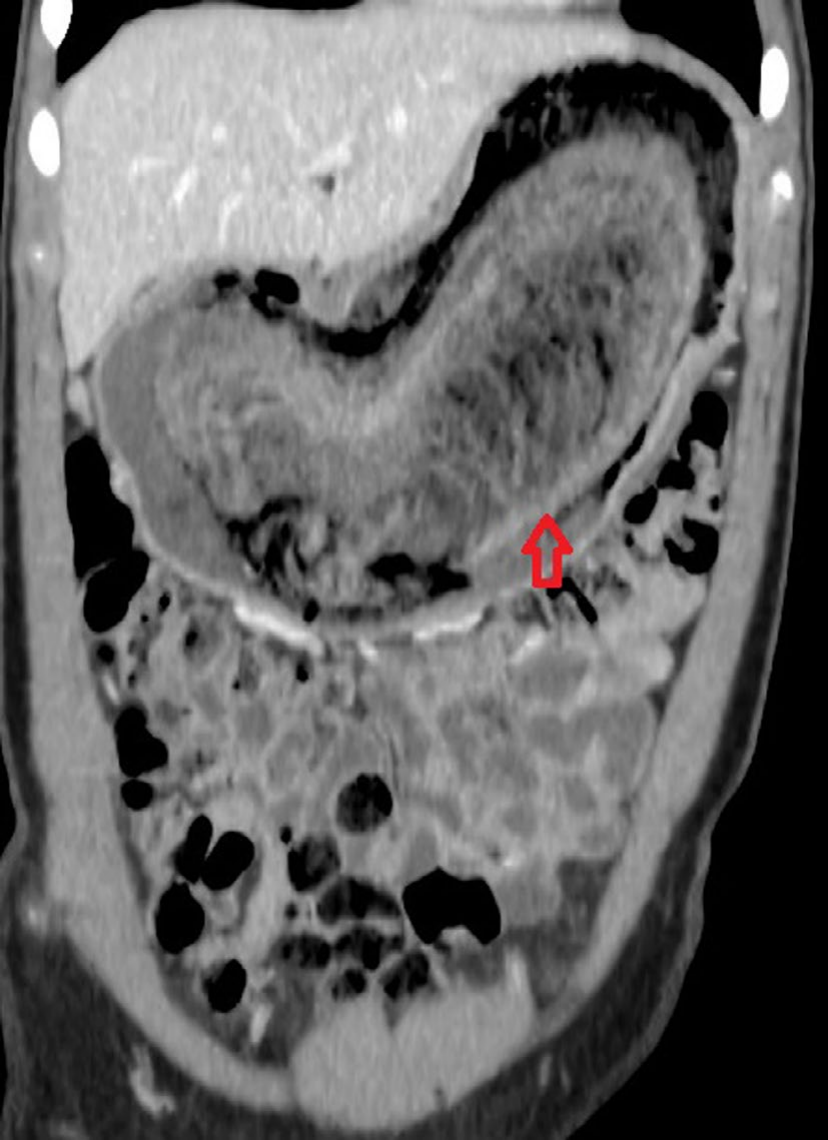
CT scan showing a low‐density intragastric mass which contains air bubbles and exhibits a characteristic mottled appearance. The arrow is demonstrating the intragastric mass
